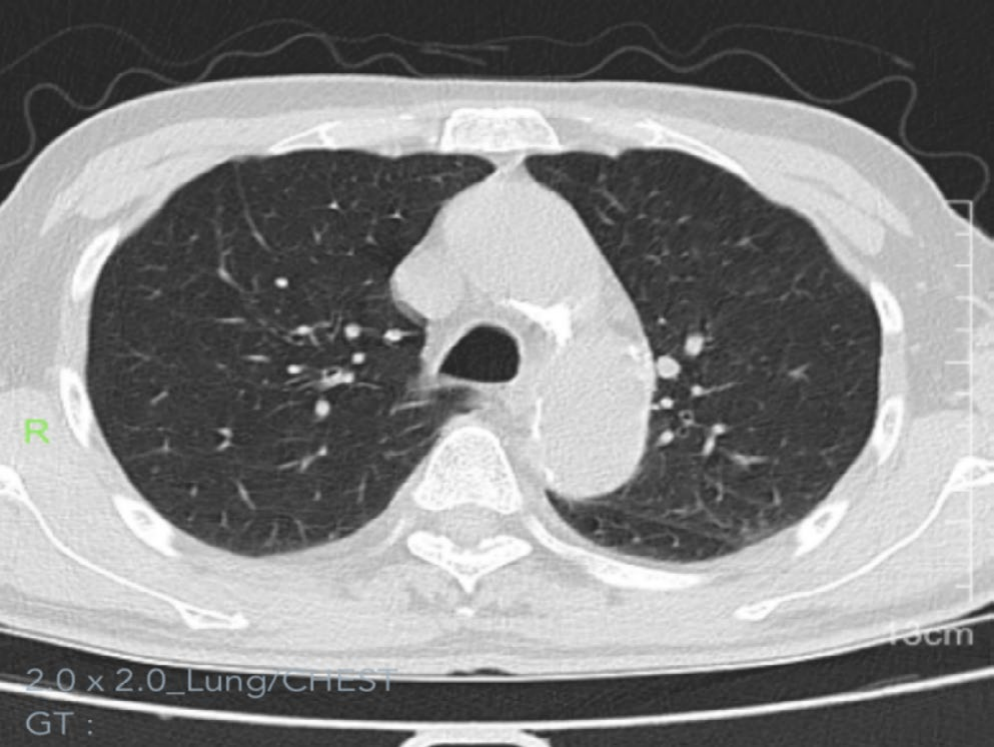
Chest CT at 1 month post‐radiotherapy: Unenhanced lung window demonstrates clear bilateral bronchovascular bundles without abnormal course or distribution.
